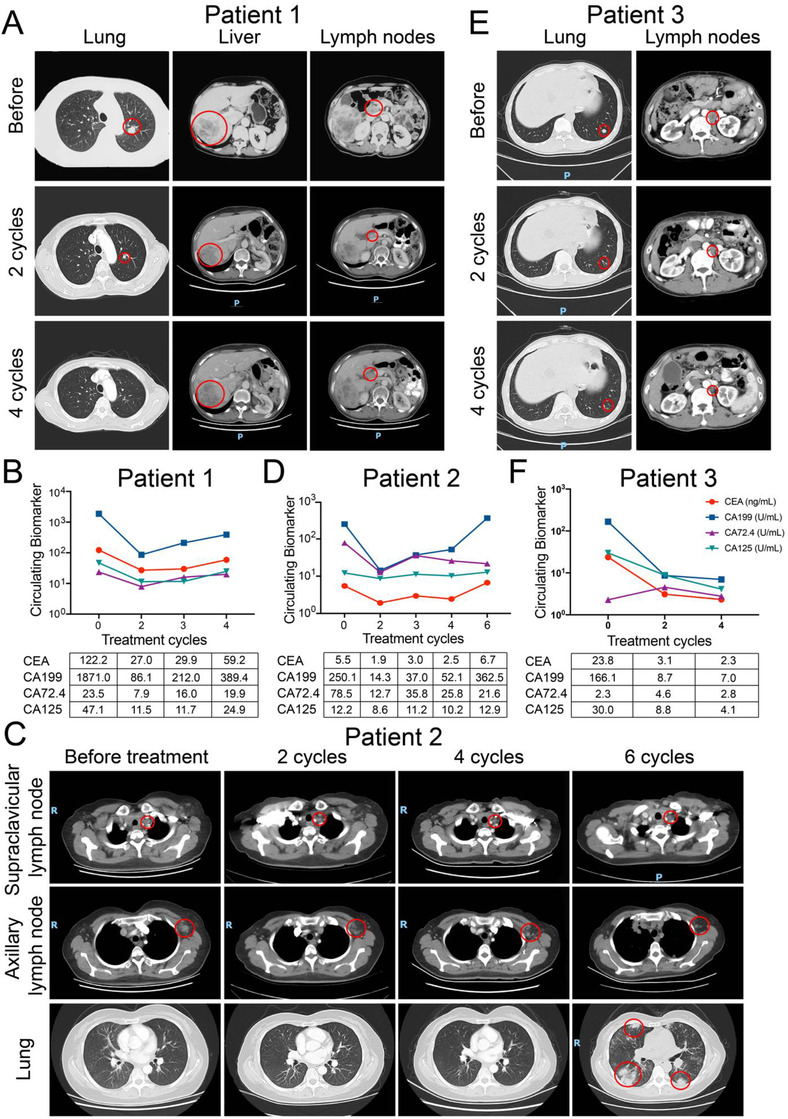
The clinical responses of three HER2‐positive GC patients treated by pyrotinib combined with SHR6390. A, CT scans of tumor metastases in lung, liver, and hepatic hilar lymph nodes during four cycles of combination treatment in patient 1. C, CT scans of tumor metastases in lung, axillary, and supraclavicular lymph nodes during six cycles of combination treatment in patient 2. E, CT scans of tumor metastases in lung and retroperitoneal lymph nodes during four cycles of combination treatment in patient 3. B, D, and F, The dynamic change of CEA, CA199, CA72.4, and CA125 during combination treatment in patient 1, 2, and 3
